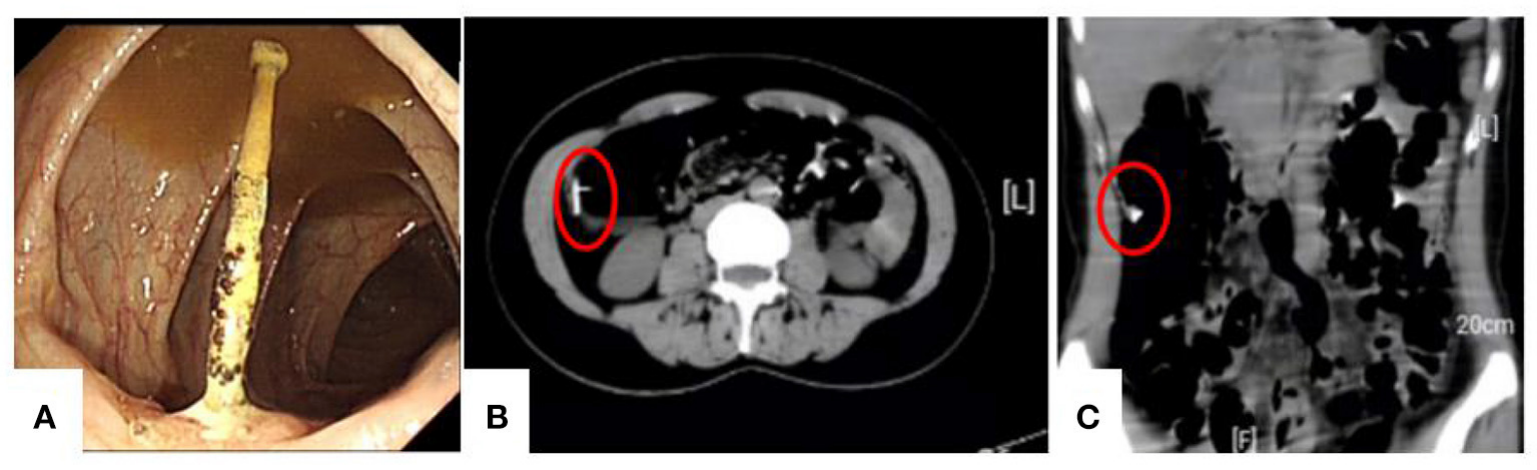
Colonoscopy and abdominal CT findings. Colonoscopy showed a foreign body similar to a nail in the ascending colon, and a local ulcer was formed (A). CT showed a foreign body through the wall of the colon (B,C).
